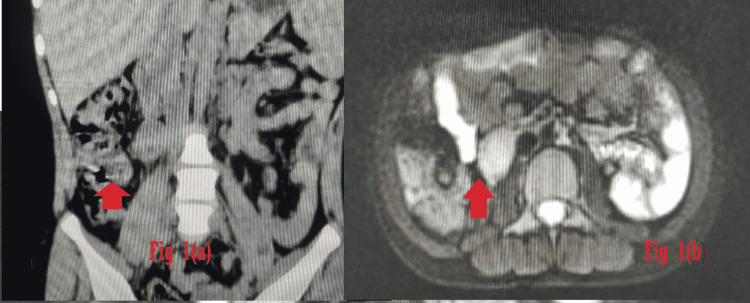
(a) MRI abdomen coronal cross-section view, (b) transverse cut section at L3 level showing hyperintense mass (2 × 1 × 3 cm) in the paracaval location in the inferior pole of the right kidney and anterior to the right psoas muscle.
